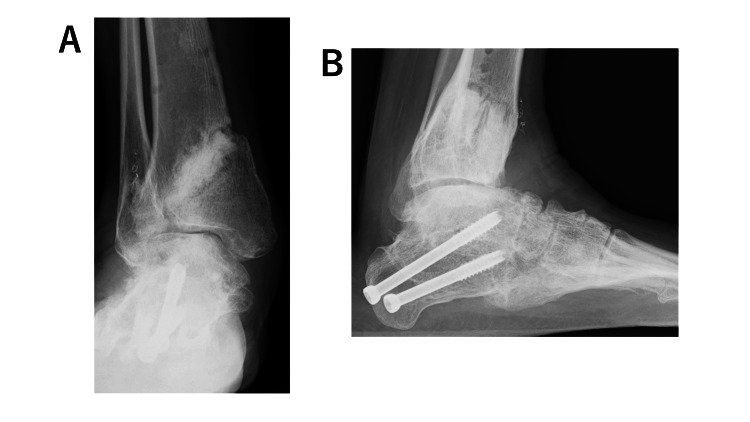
Plain radiographs of the right foot after the previous surgery. (A, B) The foot is plantigrade
